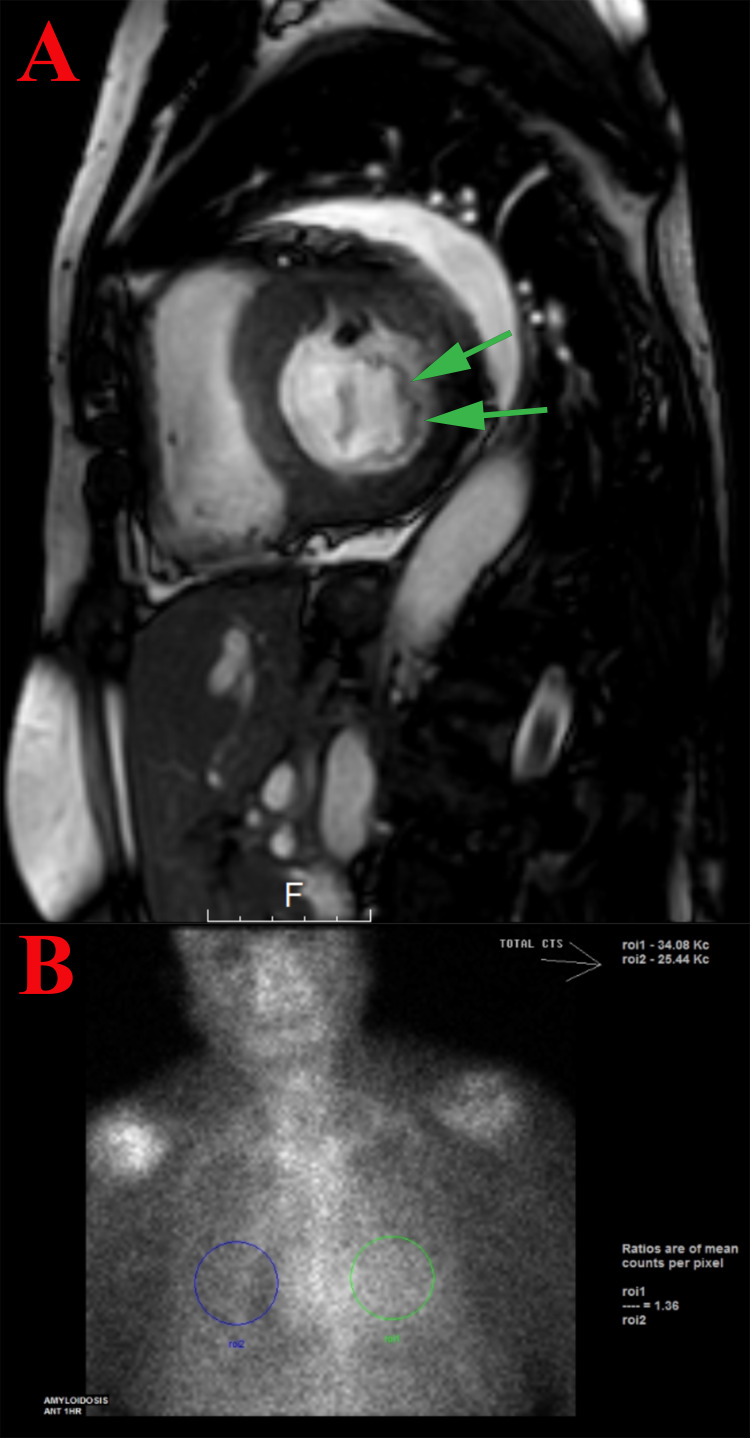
Caridiac imagings A) Cardiac magnetic resonance imaging (CMR) showed mildly increased LV thickness at the basal septal wall 13 mm with prominent trabeculations in the mid and apical segments (the green arrows). High T1 value (average 1240), which may indicate infiltrative myocardial disease. B) 99mTc-labeled pyrophosphate (PYP) cardiac scan showed features strongly suggestive of transthyretin cardiac amyloidosis with grade three of the visual interpretation (the green circle indicates the degree cardiac tissue, the blue circle indicates the degree of rib tissue).
